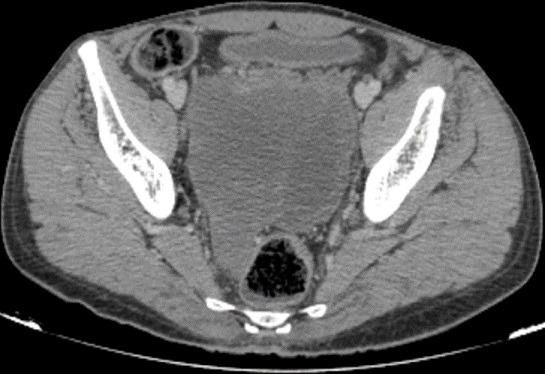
Abdominal CT scan showing rectal’s tumour size regression
